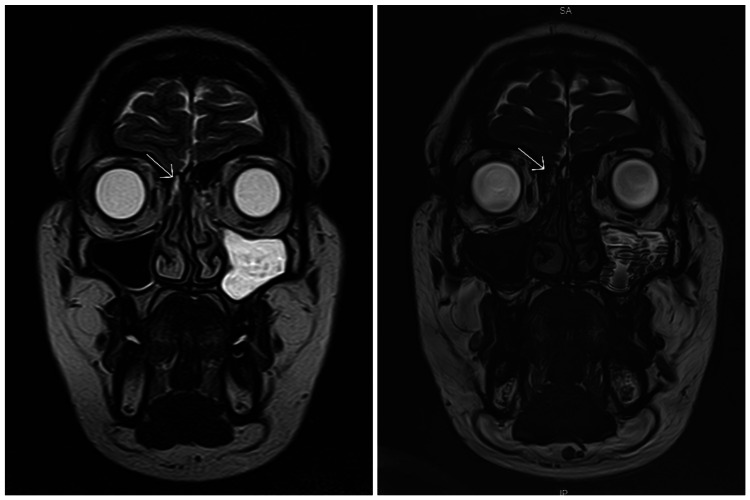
MRI (FIESTA sequence) coronal view. Asymmetric accentuated linear tract (arrow) visible in T2-weighted (left) and SSFP sequence (right) window. FIESTA, fast imaging employing steady-state acquisition; SSFP, steady-state free precession.
